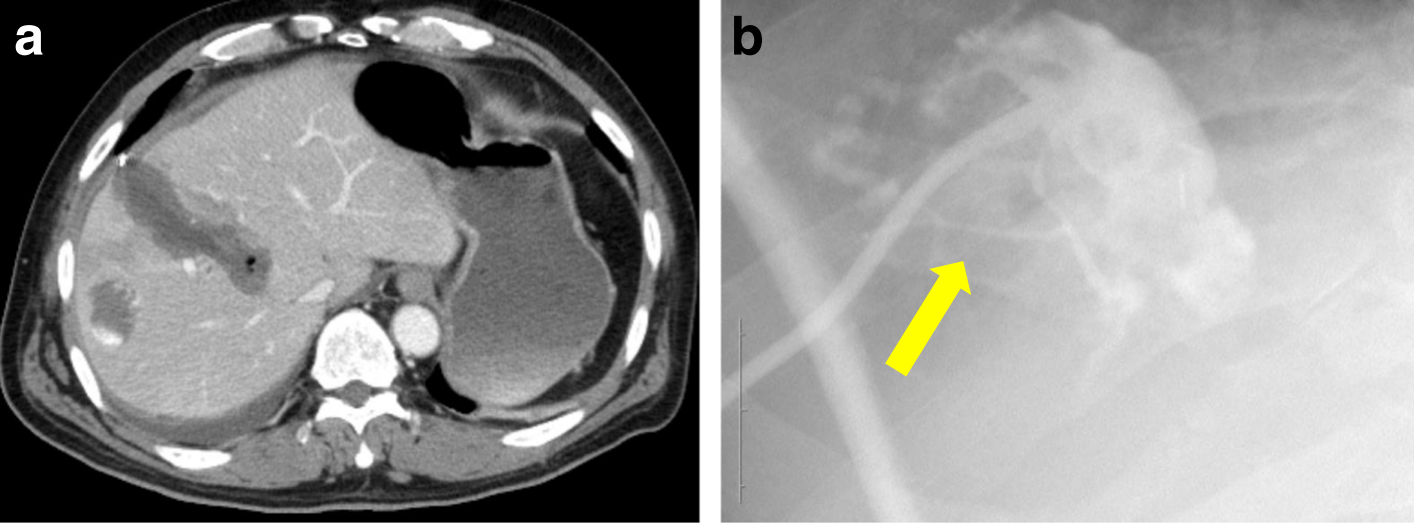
On postoperative day 19, abdominal computed tomography showed the fluid at the liver cut surface (a). Cholangiography via the c-tube did not detect the anterior branch of the bile duct (arrows) and does not communicate with the abdominal cavity (b)
